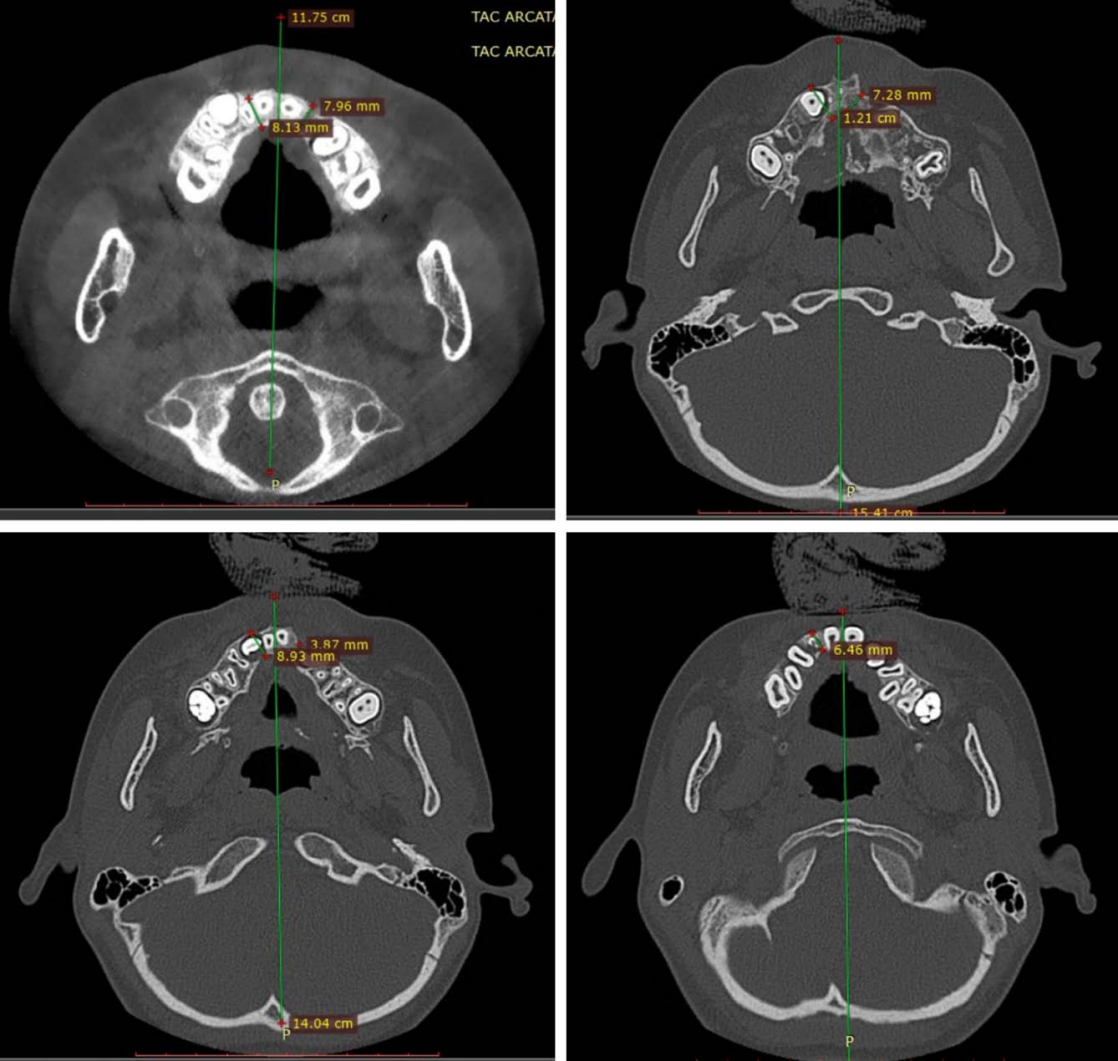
Axial CT scans show different degrees of alveolar ossification depending on the calculated ratio of the cleft versus noncleft side. (*Above*, *left*) Ideal ossification: from 100% to 75%. (*Above*, *right*) Good ossification: from 75% to 50%. (*Below*, *left*) Mediocre ossification: from 50% to 25%. (*Below*, *right*) Insufficient ossification: from 25% to 0%.
